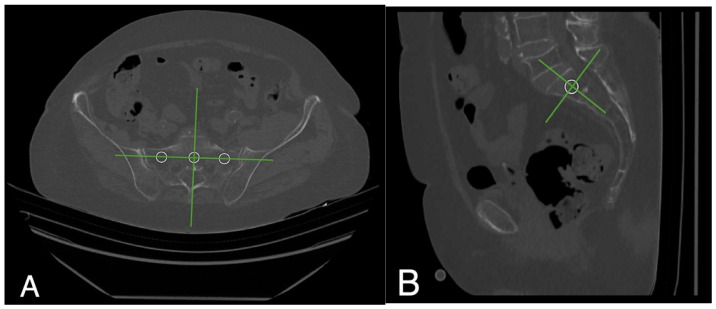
CT images of a 90-year-old female patient with a left-sided FFP IIc. (A) Axial view of the lateral fracture of the left massa lateralis; (B) sagittal view of the same fracture. The white circles represent the points to be measured at which the HU measurement is carried out. The green auxiliary lines are used for precise positioning.
